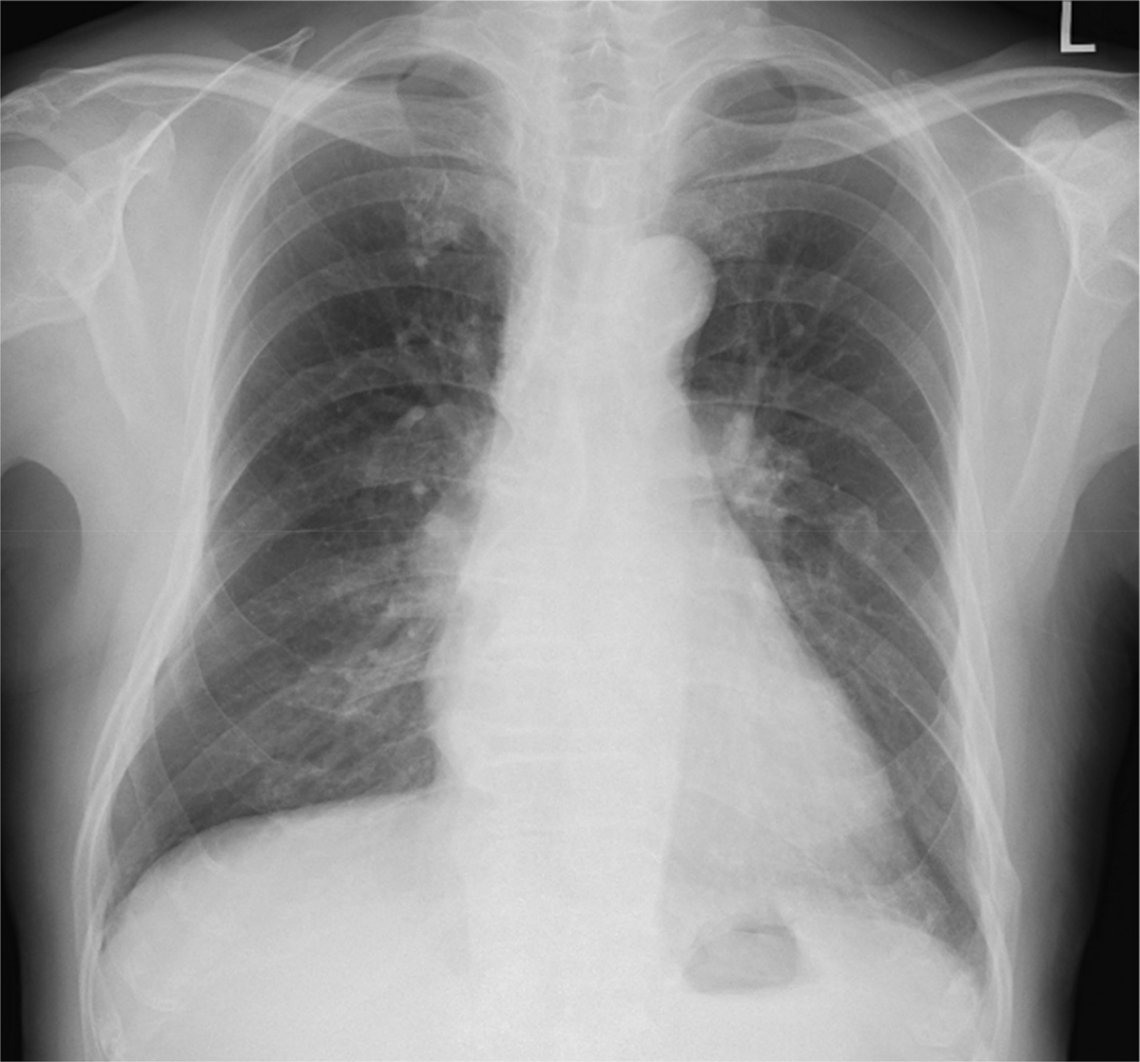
A chest radiograph showing no obvious abnormalities.
